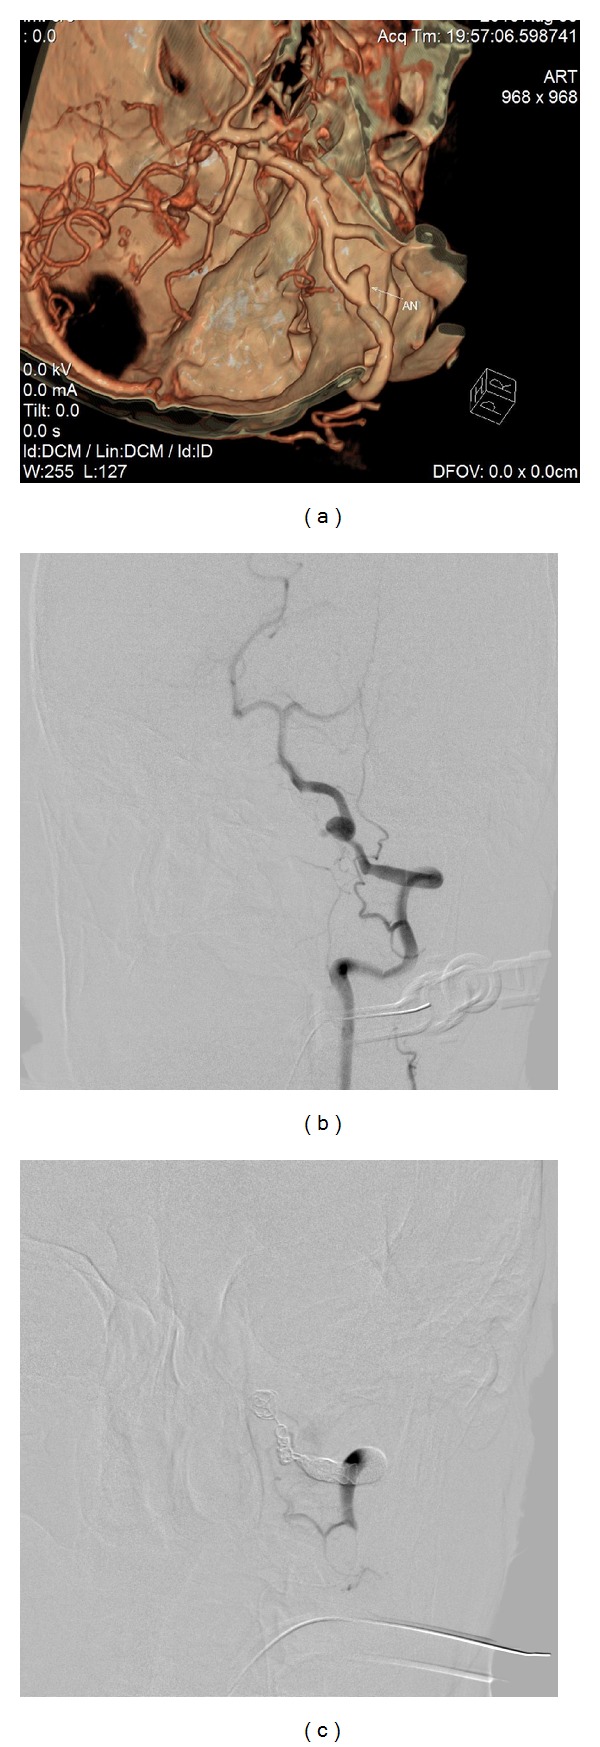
A 56-year-old hypertensive and smoker male patient presented with acute onset headache and loss of consciousness. Brain CT angiography revealed dissecting aneurysm in the distal segment of left vertebral artery. (a) This finding was confirmed by DSA. (b) The patient underwent coil embolization and parent artery occlusion. (c) Unfortunately, the patient died because of aspiration pneumonia 10 days later.
